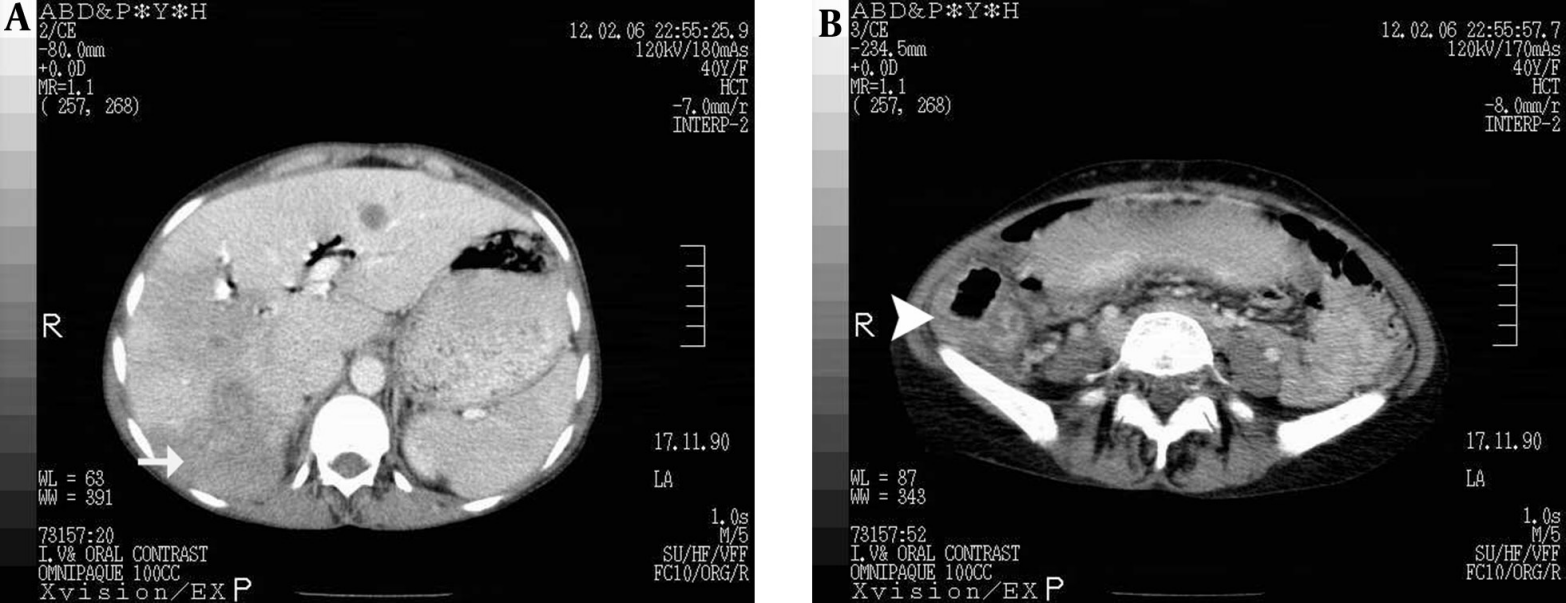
CT Scan of Abdomen A: CT Scan of the abdomen revealed hypoattenuating lesions in the liver (Arrow) and thickening of the cecal wall (Arrow head) in Figure 1 -B.
